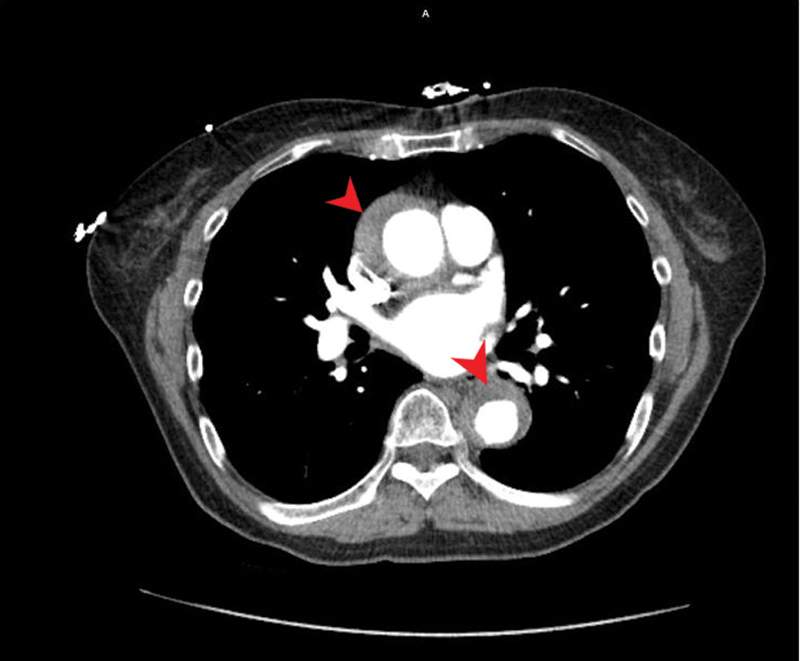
Computed tomography angiogram revealed high dense concentric mural thickening involving the ascending and descending thoracic aorta (arrows) representing an intramural hematoma.
